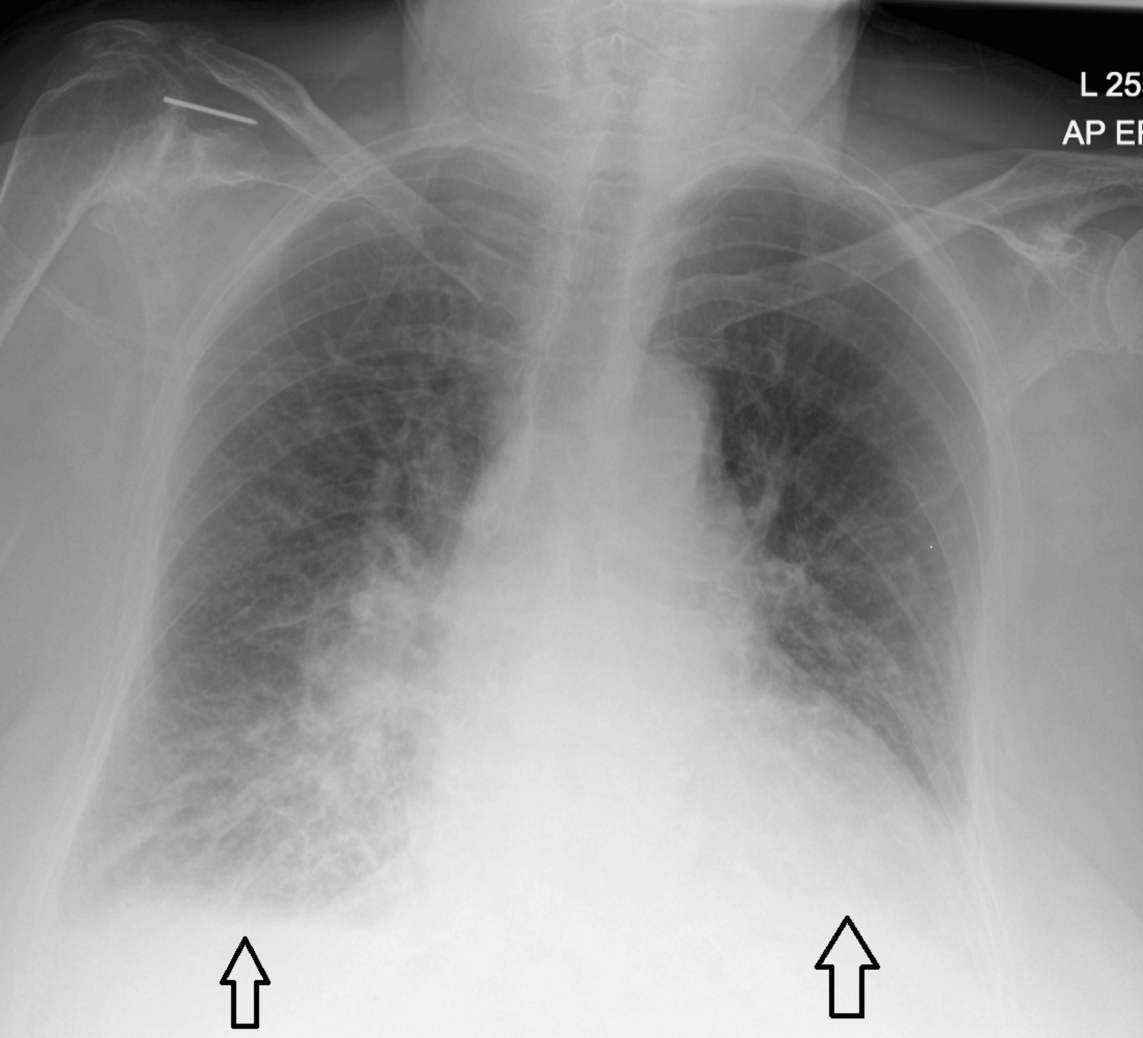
Chest X-ray on admission showing bilateral pulmonary congestion.
